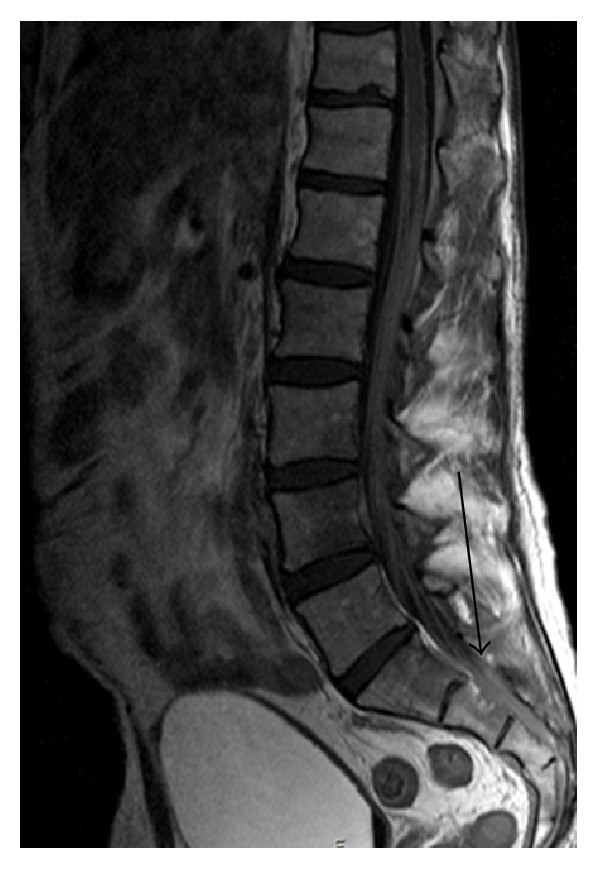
MRI lower thoracic and lumbar spine sagittal view showing enhancement of the distal spinal cord and conus medullaris.
